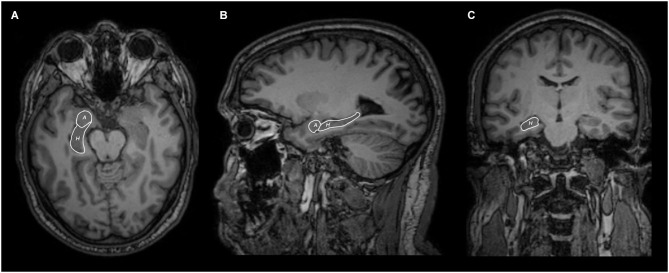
Axial (A), sagittal (B) and coronal (C) MRI views of hippocampus (H) and amygdala (A) segmentation.
